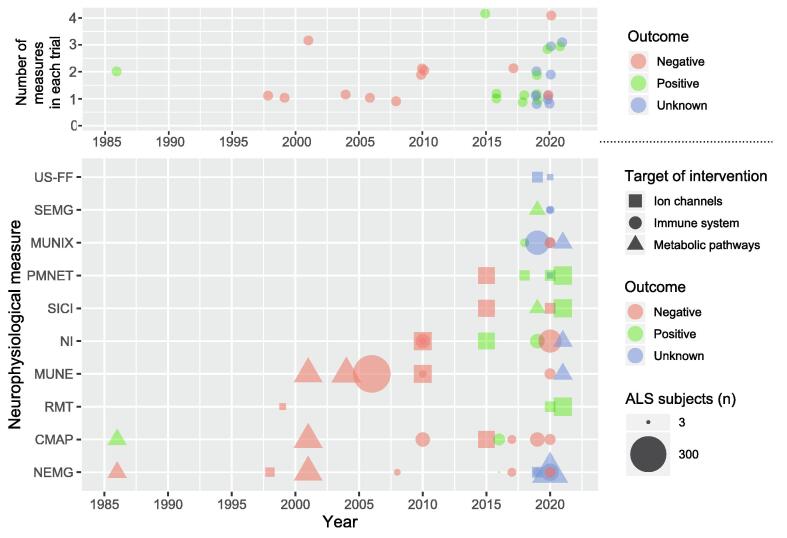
Timeline of studies. Since 1986, 32 interventional trials have employed 56 neurophysiological outcome measures in ALS subjects. CMAP, compound muscle action potential; MUNE, motor unit number estimate; MUNIX, motor unit number index; NEMG, needle electromyography; NI, neurophysiological index; PMNET, peripheral motor nerve excitability testing; RMT, resting motor threshold; SEMG, surface electromyography; SICI, short-interval intracortical inhibition; US-FF, ultrasound-fasciculation frequency.
